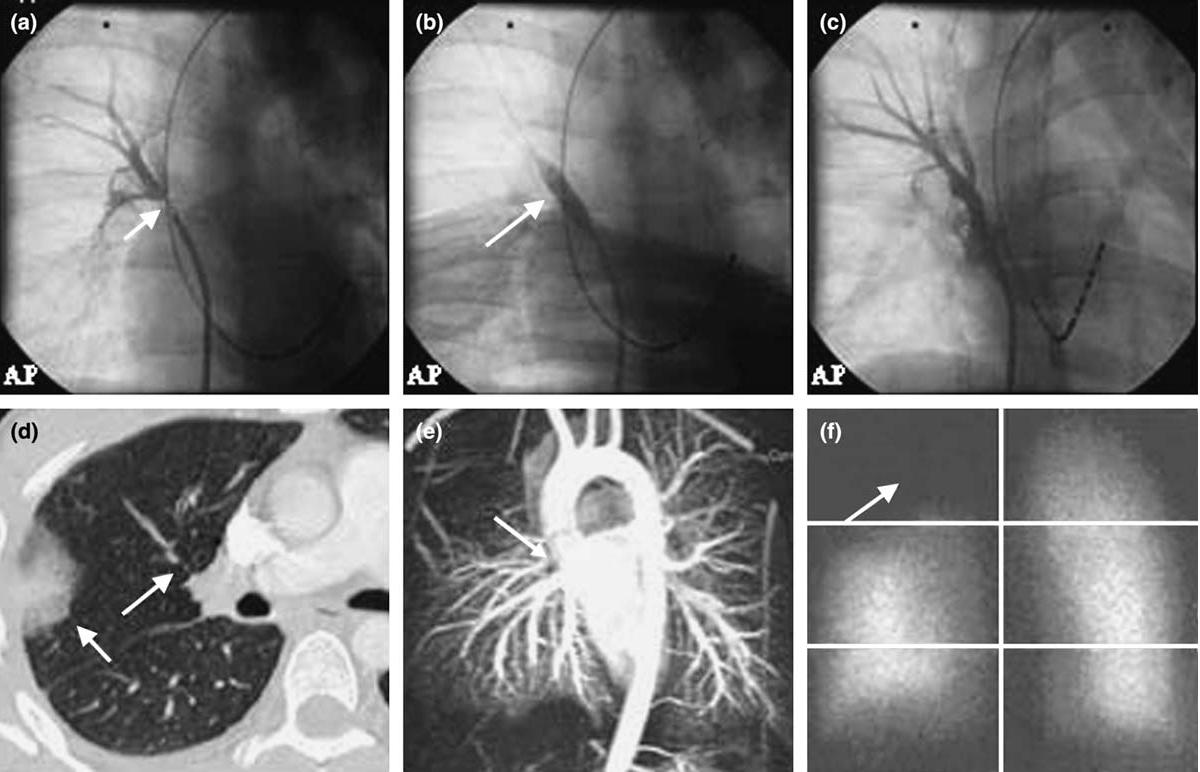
Images of a right superior pulmonary vein stenosis (Reproduced with permission from ref. [14]): (a) Angiographic view of the high grade stenosis (arrow). (b) Balloon dilatation (arrow). (c) Result after dilatation and stenting. (d) Pre-interventional computed tomographic scanning of the stenosis (arrow), including infiltration in the dependent lung lobe (arrow). (e) Three-dimensional magnetic resonance reconstruction of the stenosis (arrow). (f) Lung scan showing perfusion deficit in the right upper field (arrow).
